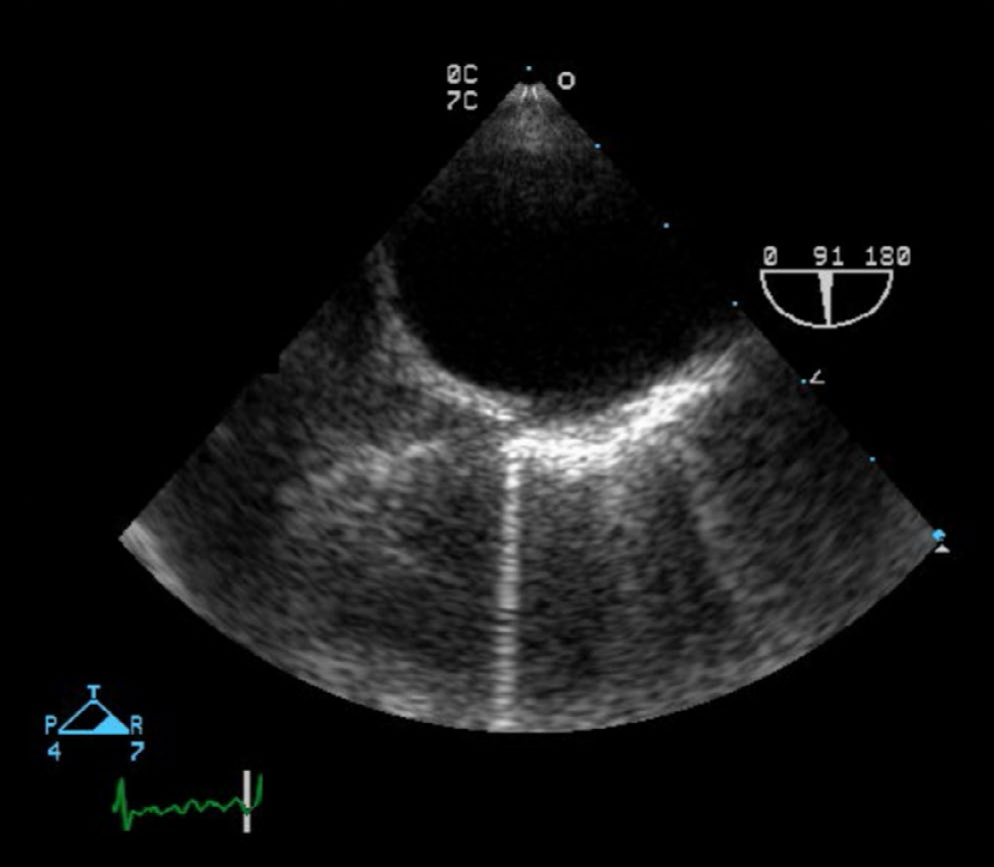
Transesophageal echocardiogram showed only minimal aortic arch atherosclerosis (Patient # 2)
